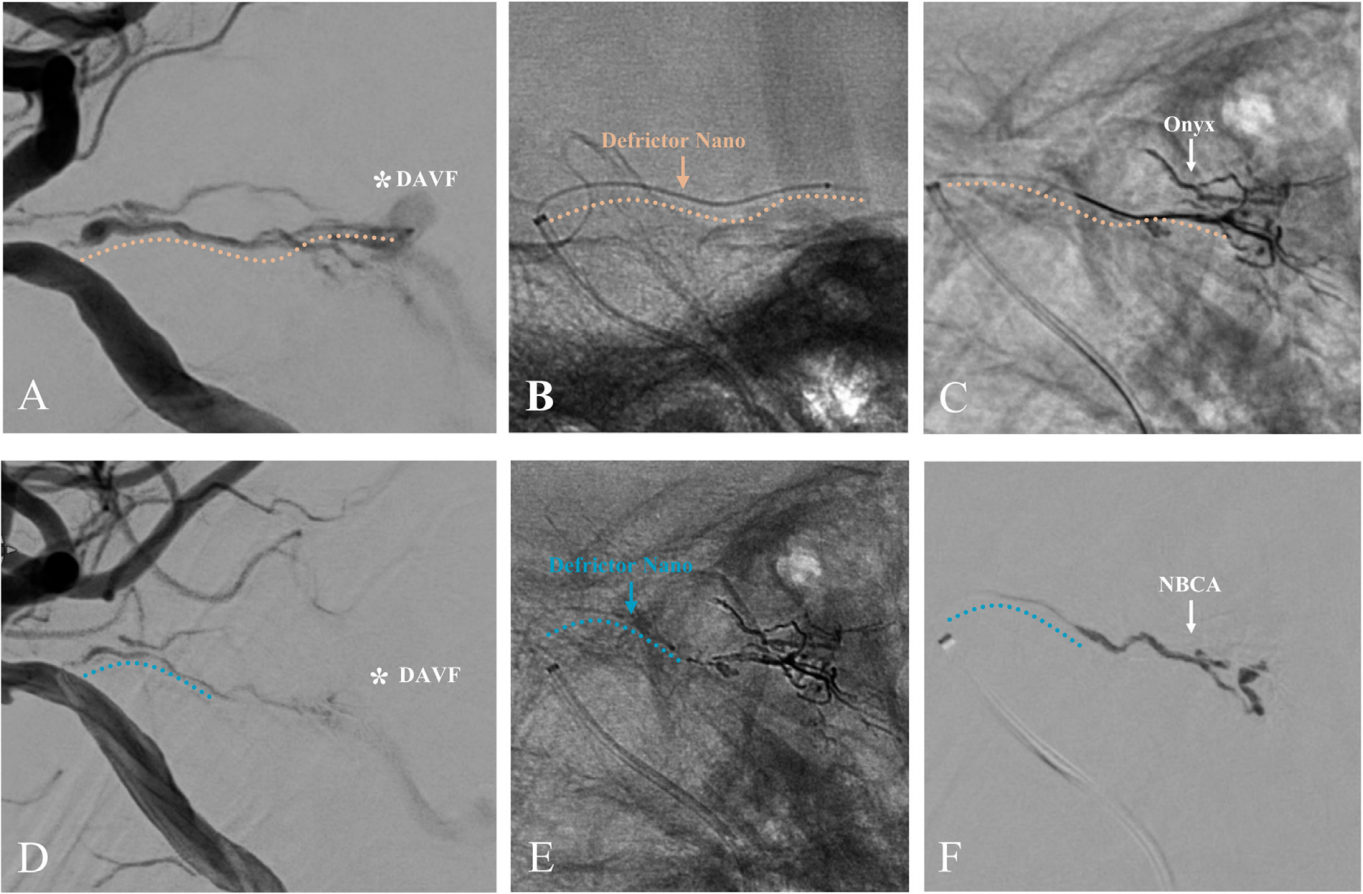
Operative images of transarterial embolization (TAE) for left tentorial dural arteriovenous fistula (TDAVF). (A,B) First embolization using Onyx: yellow line indicates the position of the microcatheter. Defrictor Nano catheter easily passes the stenotic lesion and reaches near the shunt. (C) X-ray film showing the Onyx casting. (D,E) Incomplete embolization because of the obstruction in the DeFrictor Nano. Residual DAVF is visualized (asterisk). Second embolization using n-butyl-2-cyanoacrylate (NBCA): blue line indicates the position of the microcatheter. Reapproach via the same feeder as the first embolization. We are forced to inject from the more proximal site because the area near the shunt is already filled with Onyx. Defrictor Nano reaches near the already-filled Onyx cast. (F) Superselective angiogram via a DeFrictor Nano showing that NBCA filling with the residual shunt. No significant distal dispersal or proximal regurgitation of NBCA is observed.
